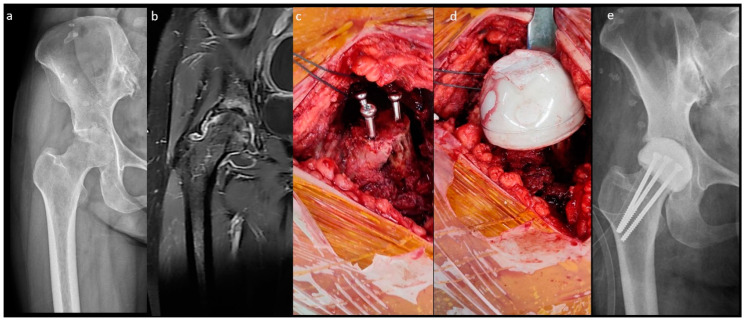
Medullary-sparing (MS) spacers. (a) Pre-operative radiograph showing diffuse cartilage destruction, (b) pre-operative MRI showing diffuse fluid accumulation within the joint, (c) three endoskeletal screws inserted to the femoral neck, (d) fabrication of the MS spacer using the bulb-shaped mold, and (e) post-operative radiograph with the MS spacer in situ.
